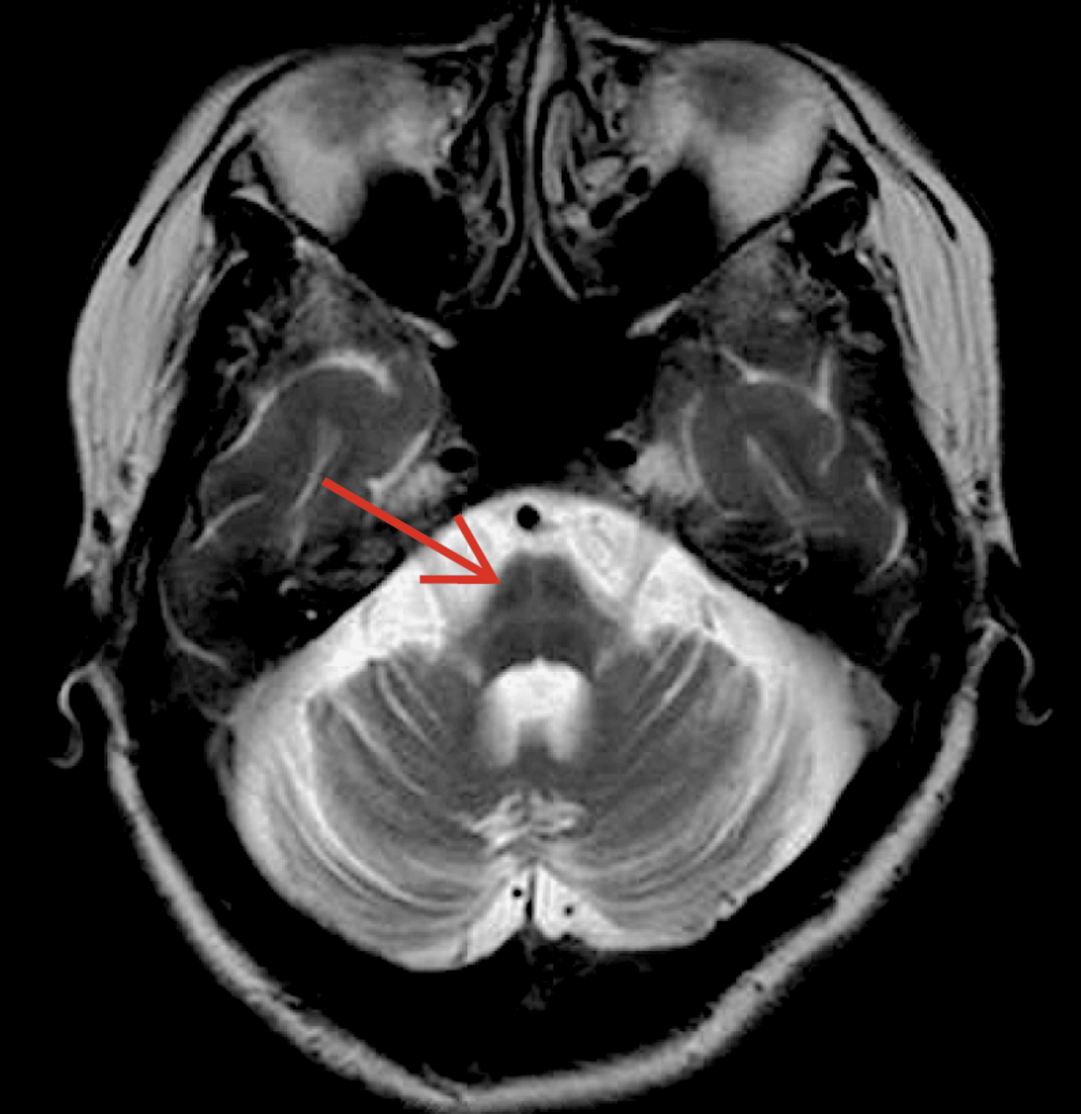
Cruciform pontine hyperintensity ("hot-cross bun" sign) seen in patients with MSA on cranial MRI (axial view) MSA - multiple system atrophy; MRI - magnetic resonance imaging
